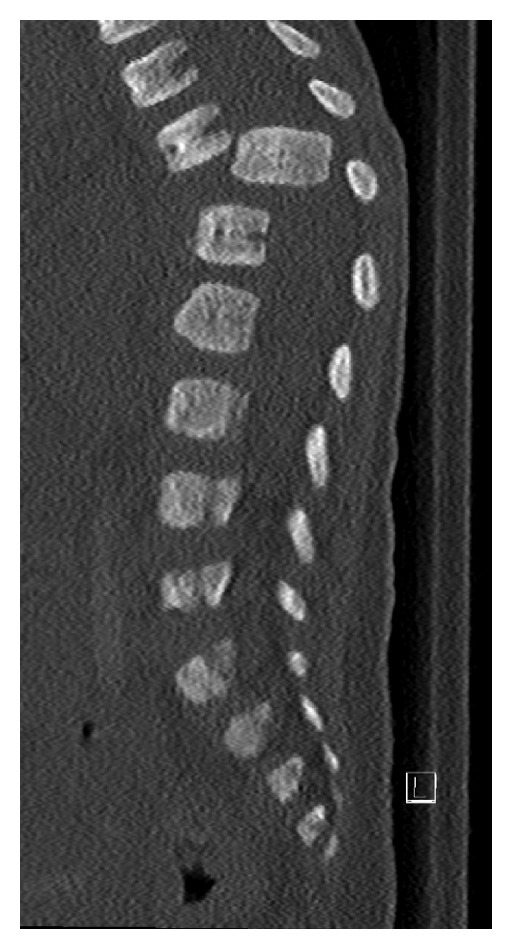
CT of the spine demonstrating complete dislocation of T11 and T12 vertebral bodies. The T12 vertebral body appears to be rotated counterclockwise on axial image and displaced posteriorly into the bony spinal canal, resulting in severe spinal canal stenosis.
